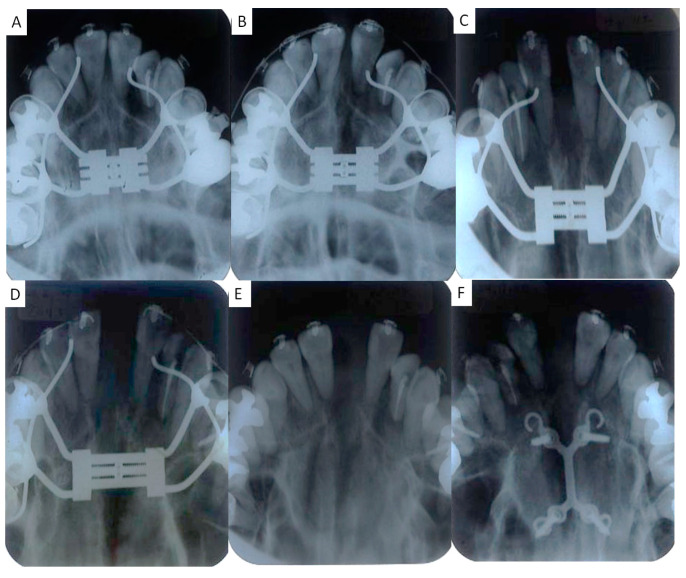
Occlusal radiographs. (A) Before SARME; (B) Day 6 after SARME; (C) Day 11 after SARME; (D) Day 25 after SARME (Completion of maxillary expansion); (E) Day 48 after SARME (immediately after removal of Hyrax appliance); (F) Day 48 after SARME.
